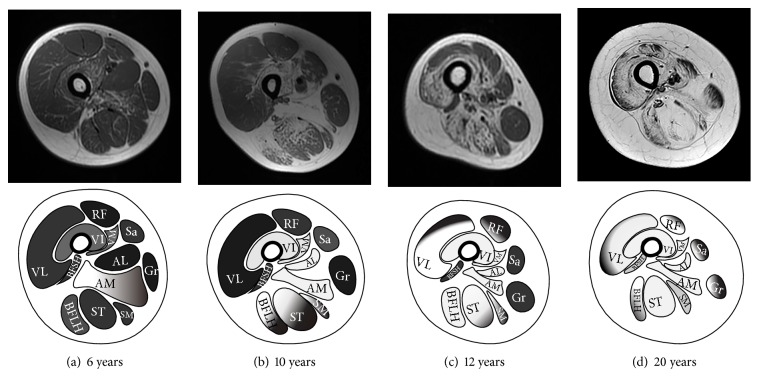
Axial T1-weighted images of the right thigh for (a) patient 3, (b) patient 4, (c) patient 8, and (d) patient 10 at the level of the mid-femoral diaphysis showing initial fatty infiltration of the posterior thigh muscles with gradual progression anteriorly as the disease progressed.
